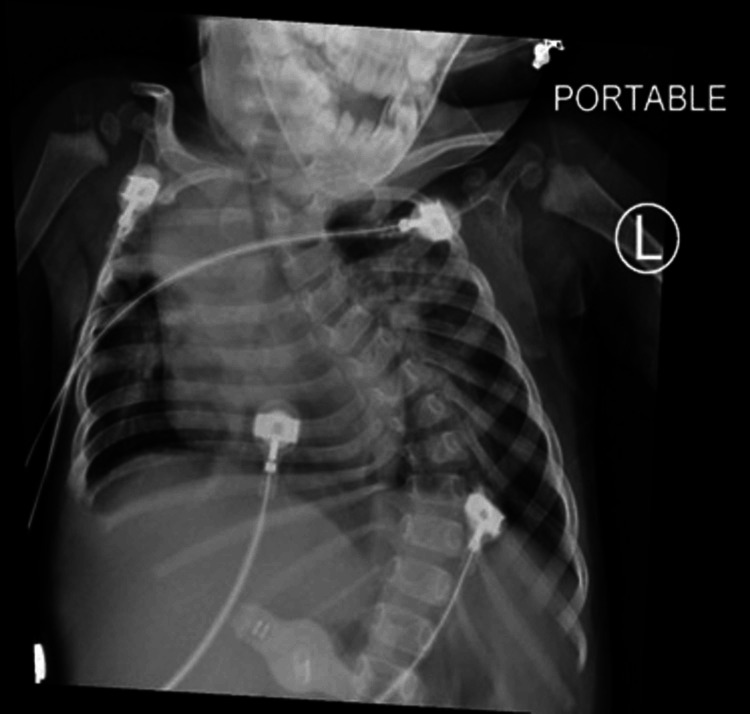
Chest X-ray, portable of a patient with Birk-Barrel Syndrome Bilateral perihilar opacities with right upper lobe atelectasis. Abnormal curvature of spine consistent with severe dextroscoliosis.
